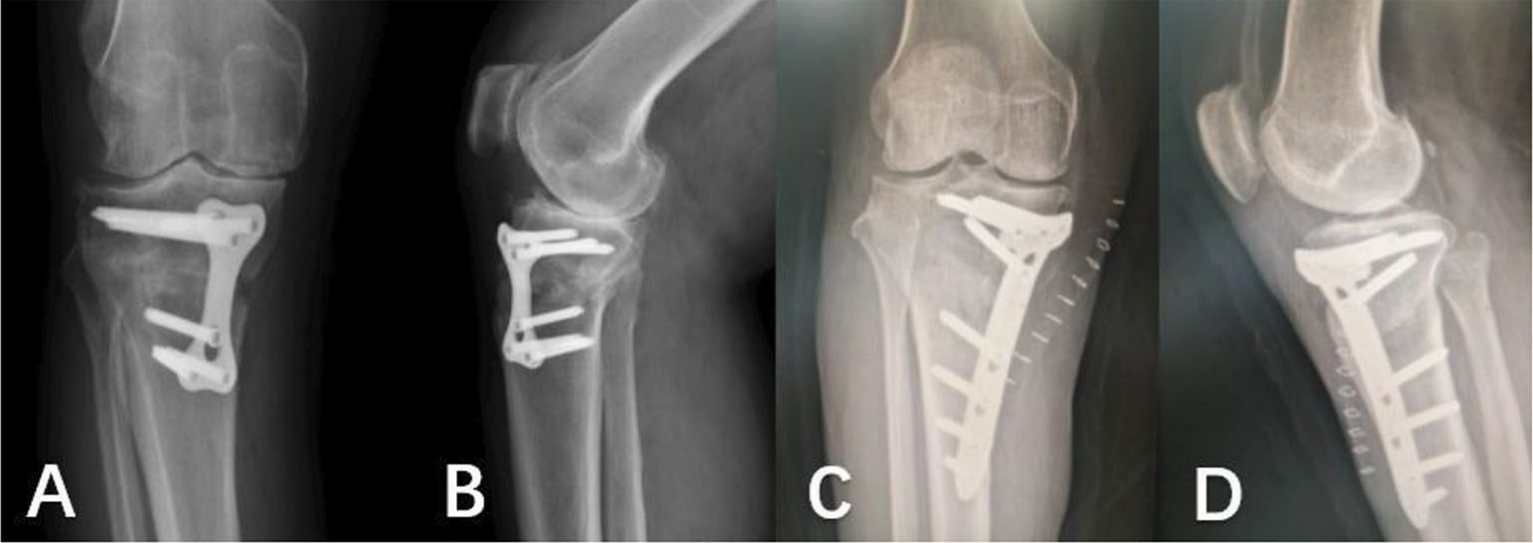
A, B: DT-LCP frontal and lateral X-rays; C, D: T-LCP frontal and lateral X-rays
